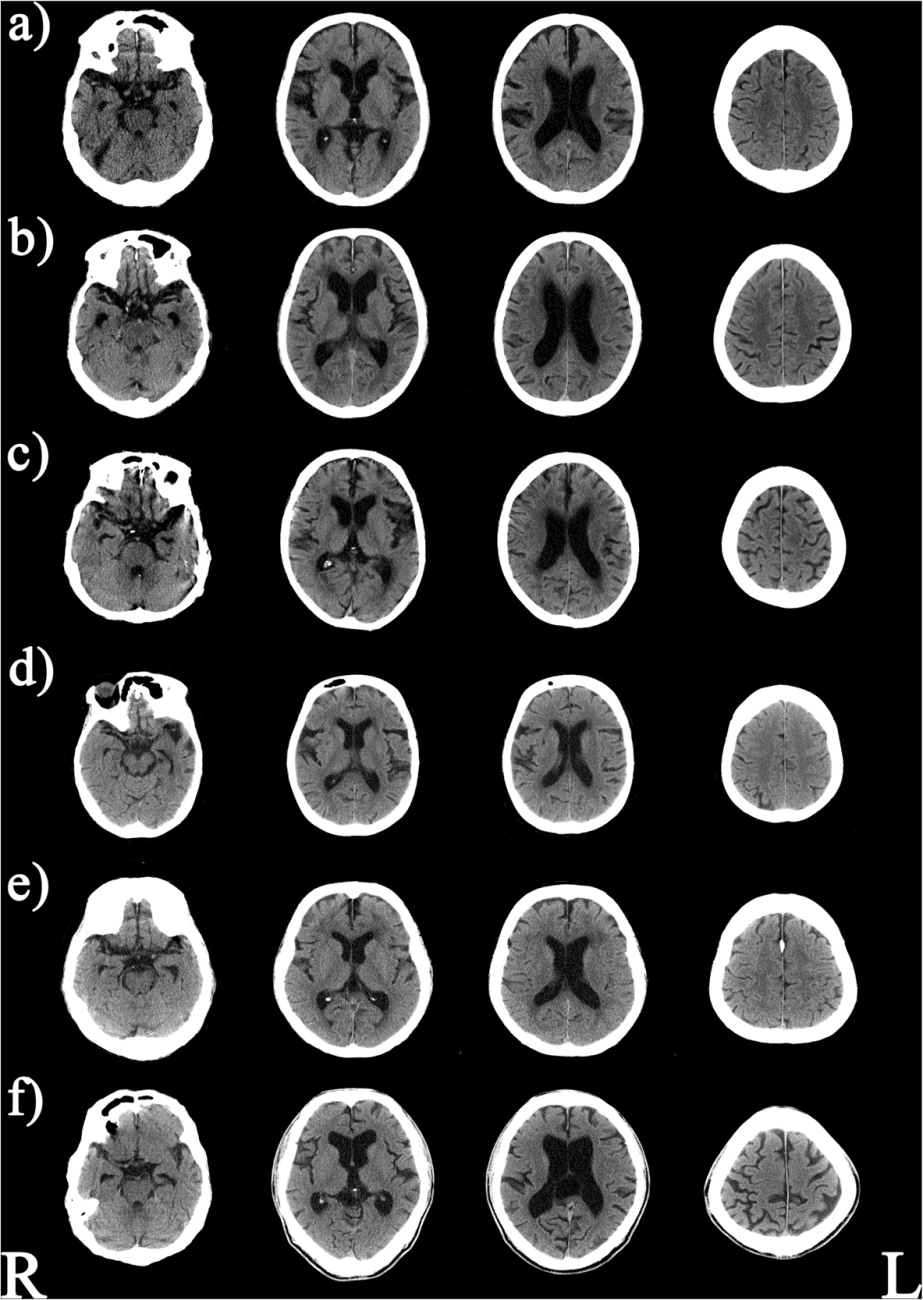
Shown are computed tomography images of a an 84-year-old male; b a 78-year-old male; c a 74-year-old male; d a 64-year-old female; and e a 61-year-old male with idiopathic normal pressure hydrocephalus. Panel f shows a computed tomography image of a 62-year-old male with secondary normal pressure hydrocephalus with a mass in the lateral ventricle. All subjects have features of an enlarged Sylvian fissure associated with ventriculomegaly and compression of high convexity. Several subjects (a, b, d, and e) have disproportionately enlarged subarachnoid space hydrocephalus findings
